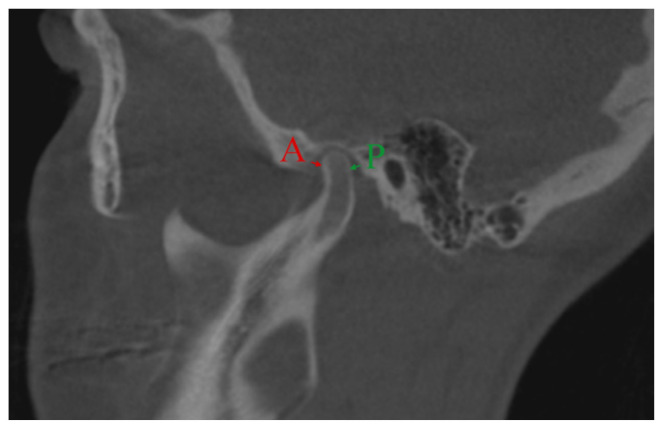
Linear measurements of the joint spaces on a sagittal cone-beam computed tomography (CBCT) multiplanar reformatted (MPR) image. Anterior (A) and posterior (P) joint spaces were measured between the most anterior and most posterior condylar head points to the glenoid fossa.
